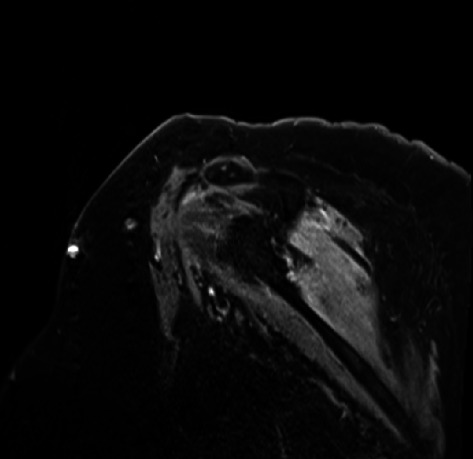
T1 MRI of the right brachial plexus reveals mild increased enhancement in the shoulder musculature.
